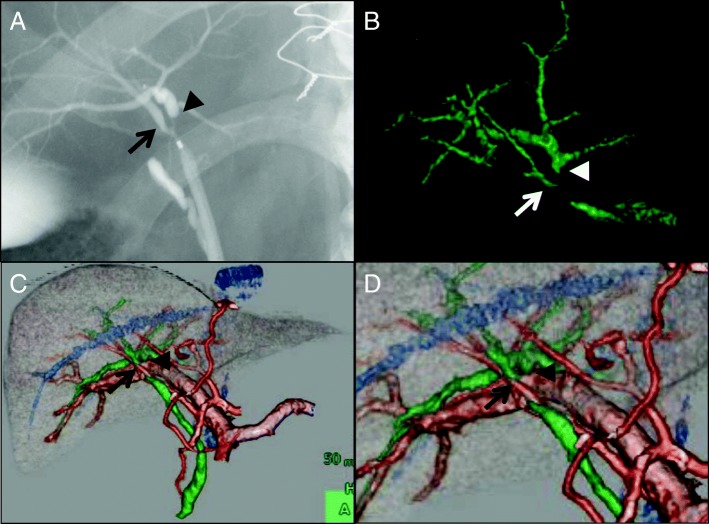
Endoscopic retrograde cholangiography (a) and 3-dimensional CT cholangiography (b, c, d) reveals stenosis of the common hepatic duct that was 20 mm in length including the right and left hepatic ducts, with involvement of the right anterior (arrow) and posterior (arrow head) bile duct bifurcation. The pattern of confluence of the right posterior hepatic artery is of the supra-portal type. The left hepatic duct is completely occluded by cancer and could not be identified
